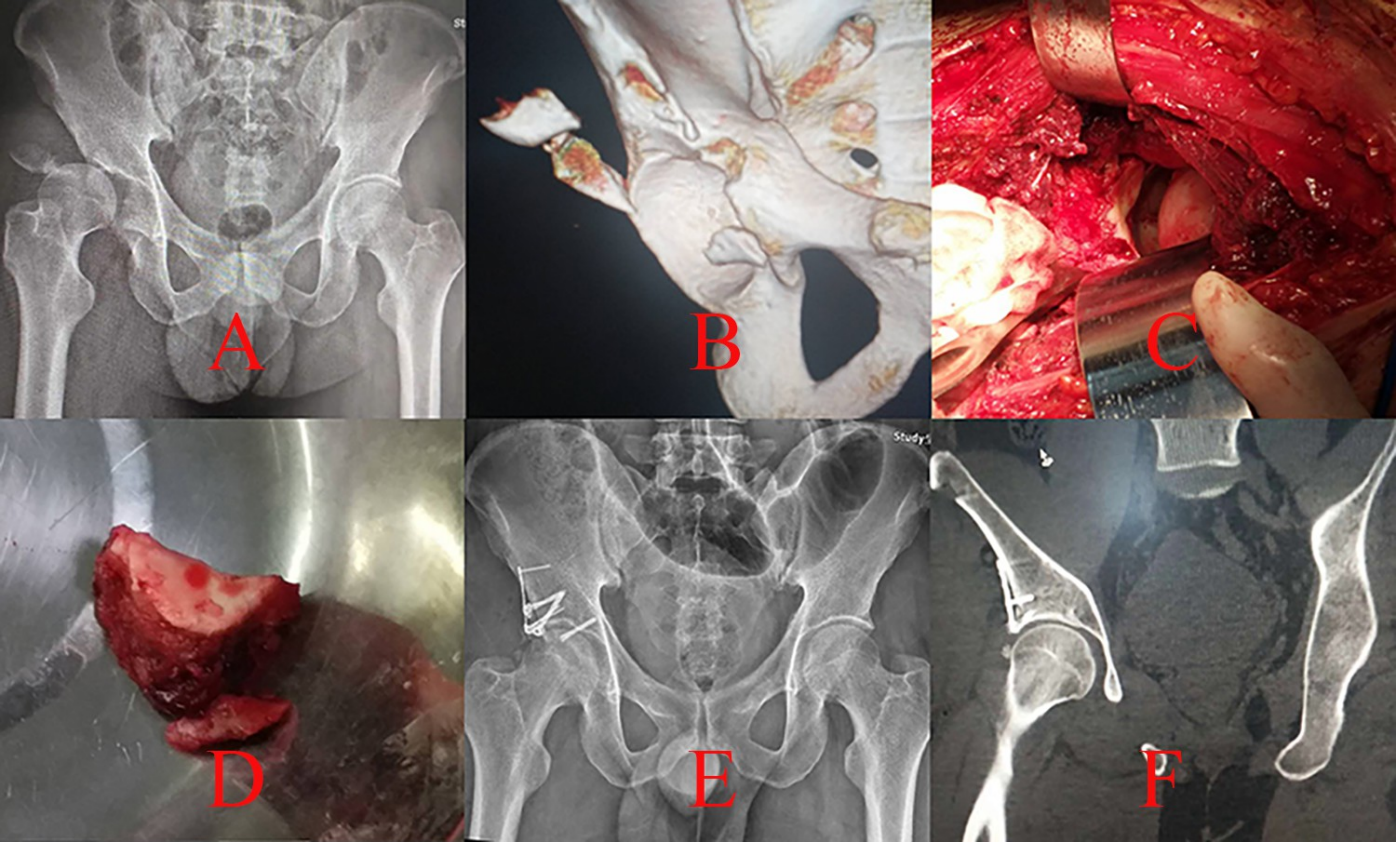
A case with A3.1 injury treated through the iliofemoral technique was presented. A, cephalad displaced femoral head and displaced roof wall fragment in AP view; B, comminuted small roof wall fragments on 3D reconstruction; C, intraoperative view (femoral head and fragments) of iliofemoral procedure; D, displaced roof wall fragment; E, plating with cannulated screws fixation presented and the reduction quality was excellent; F, excellent concentric reduction on postoperative CT scan.
